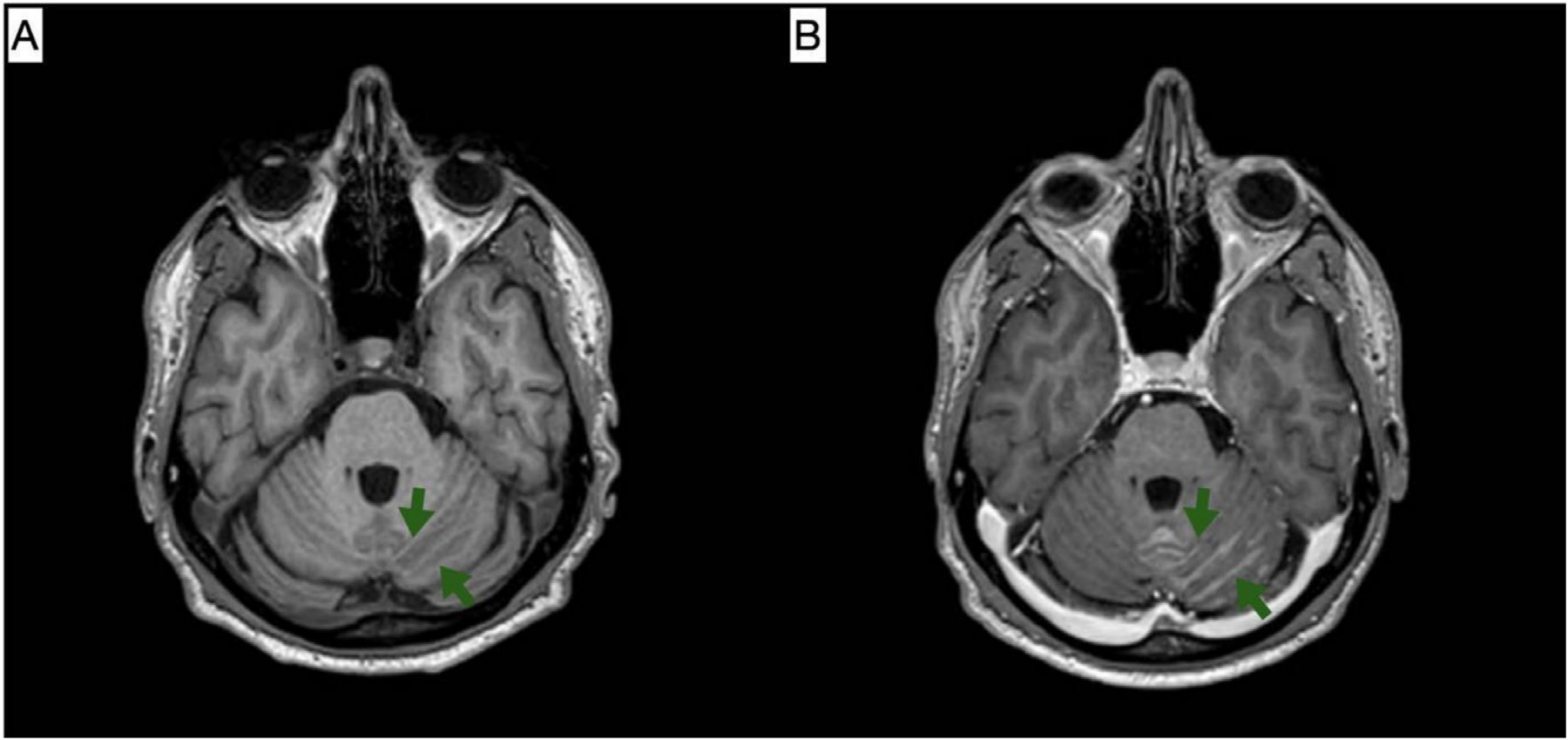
(A) T1-weighted image; (B) T1-weighted image post-contrast. Selected MRI images at the level of the superior cerebellum demonstrate leptomeningeal enhancement of the left cerebellum (indicated by green arrows), which is suggestive of metastatic deposits.
